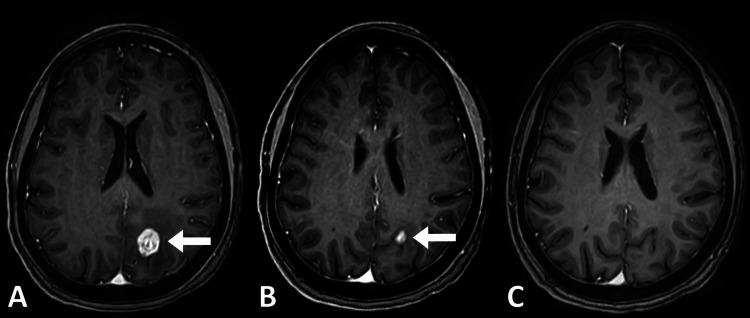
Patient treated with SRS for breast cancer metastasis. MRI C+ before (A), at six months (B), and at 12 months of treatment (C). Total regression of the lesion is observed. (Arrows: lesion). SRS: Stereotactic radiosurgery.
